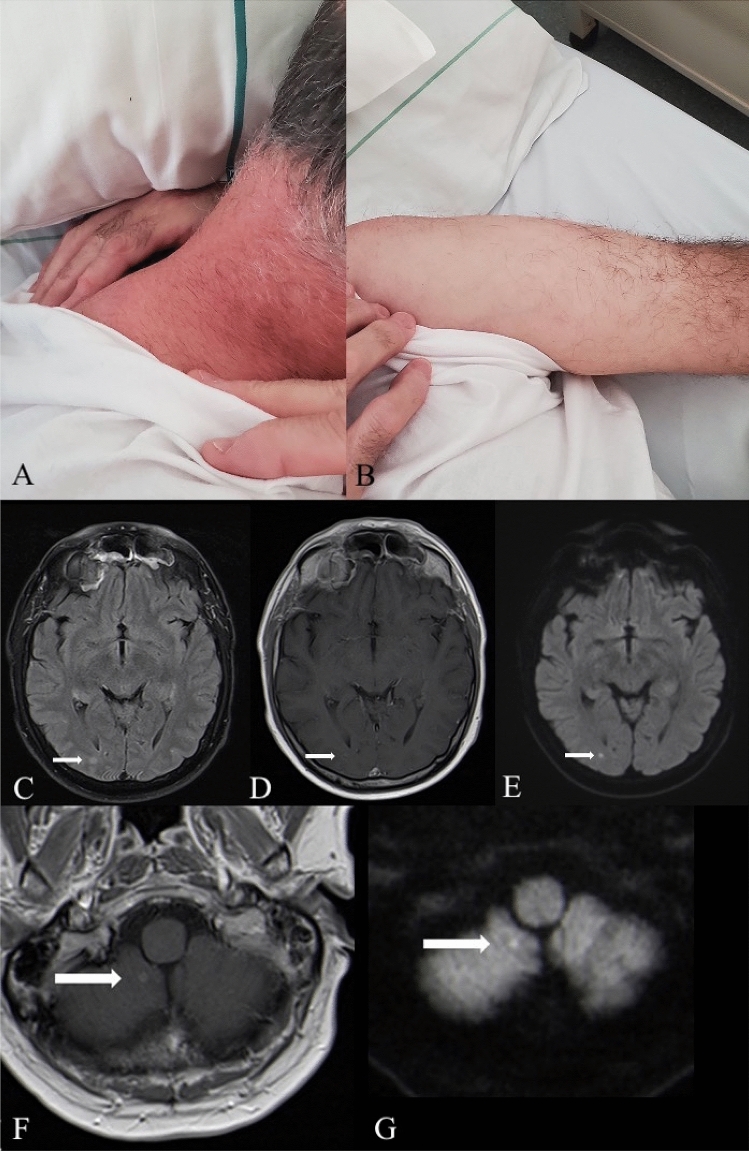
Cutaneous and intracranial lesions (A–G). A shows cutaneous involvement with nodular bulging of the nuchal region (A) and (B) upper left arm. C–E shows images of the performed MRI examination of the neurocranium on a 1.5 Tesla scanner. (MAGNETOM Solar, Siemens, Erlangen, Germany). The panel shows hyperintense signal in the TIRM sequence (C), annular contrast enhancement in contrast enhanced T1-weighted sequences (D) and diffusion restriction in diffusion weighted sequence (E). A small cerebellar lesion is shown in the posterior cerebellar lobe of the right hemisphere in contrast enhanced (F) and diffusion weighted (G) images
